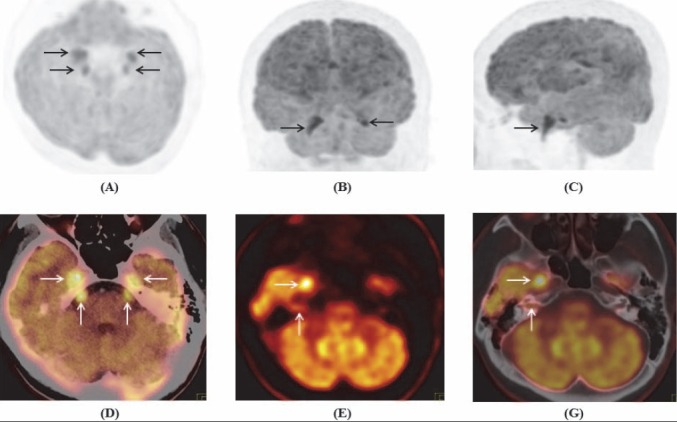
Maximum intensity projection of brain tranaxial (A) coronal (B) and sagital (C); PET/CT (D, G) and PET images (E) showed increased FDG uptake in bilateral cranial nerve V, Gasser’s ganglions, and complex of the right cranial nerve VII/VIII
